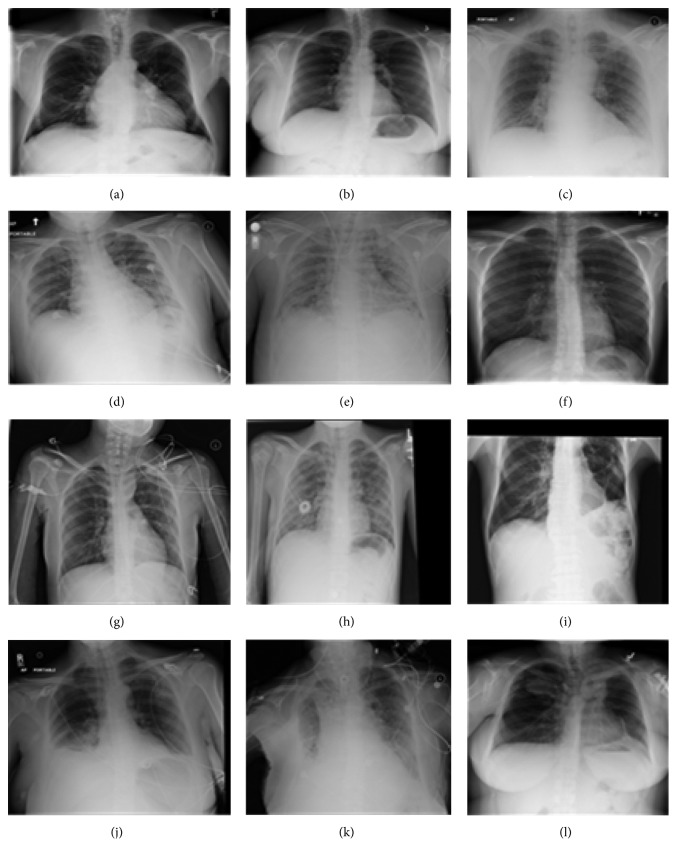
Chest X-ray diseases. (a) Atelectasis. (b) Cardiomegaly. (c) Consolidation. (d) Edema. (e) Effusion. (f) Emphysema. (g) Fibrosis. (h) Infiltration. (i) Mass. (j) Nodule. (k) Pneumonia. (l) Pneumothorax.
